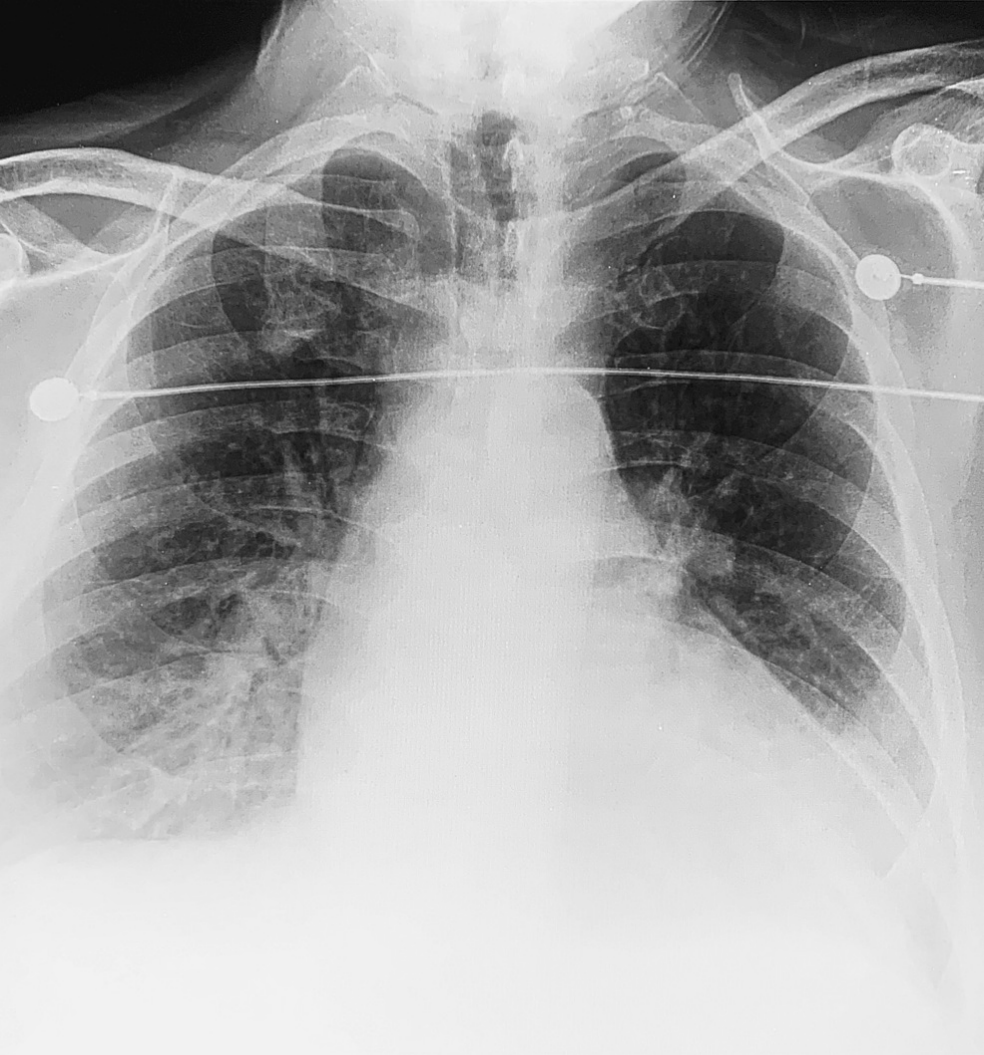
Chest X-ray demonstrating bilateral pulmonary edema and cardiomegaly supportive of CHF. CHF: Congestive heart failure.
